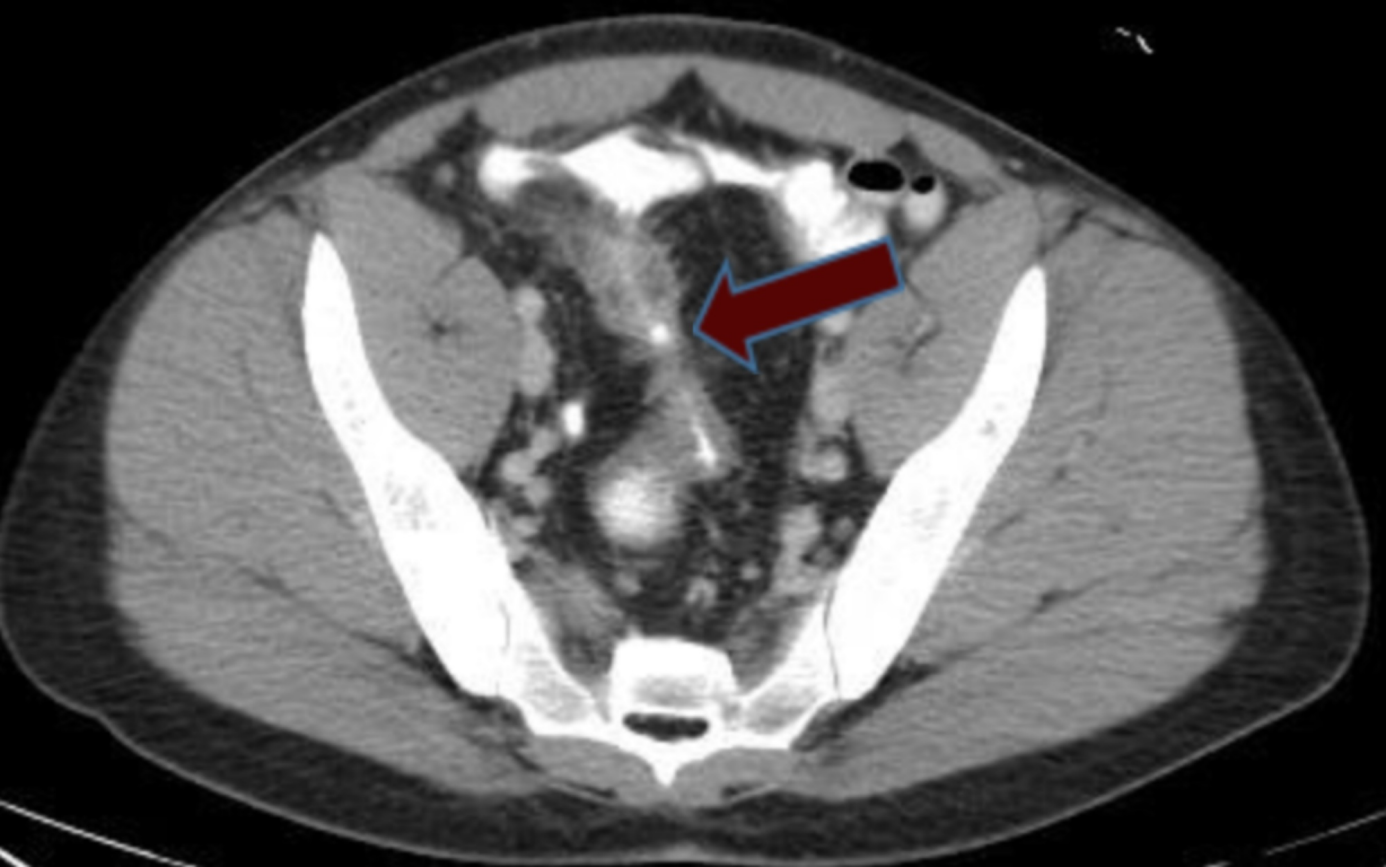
CT scan showing presence of fistula between the bladder and terminal Ileum indicated by the red arrow
